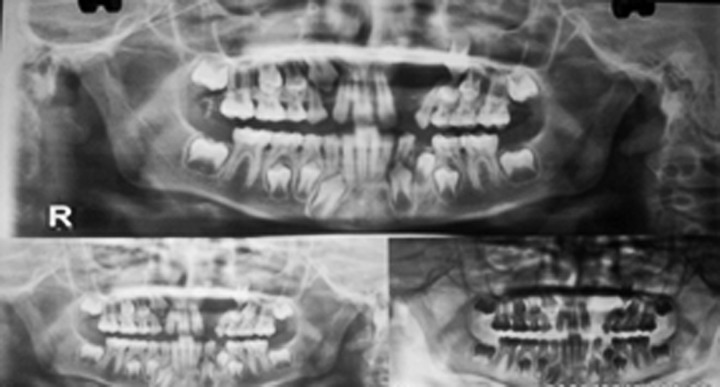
Orthopantomograph showing location of the cyst
